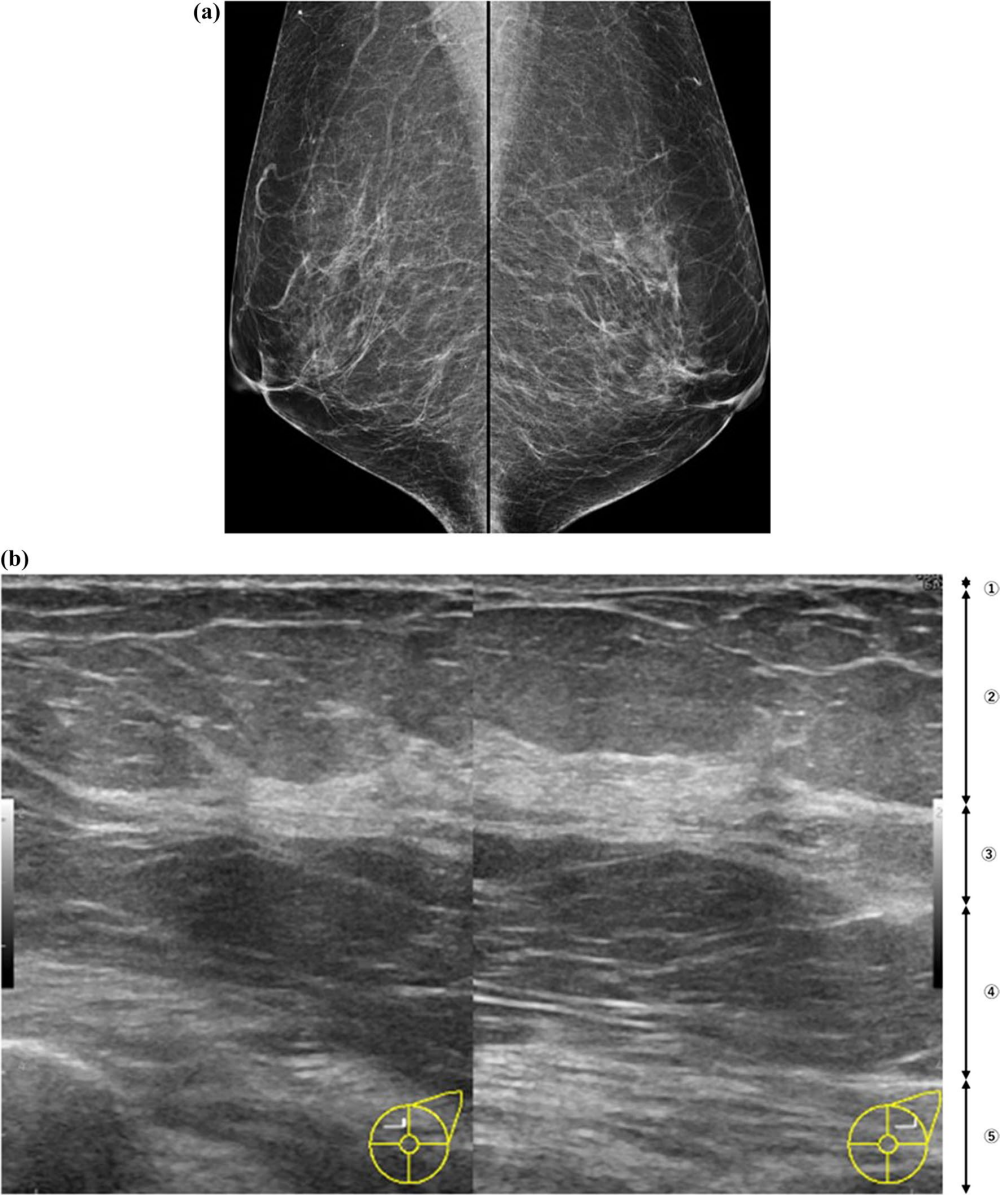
Mammographic nondense breasts that are also ultrasonographic nondense breasts without evident fat lobules in the fibroglandular tissue but with a FGT thickness-to-FAT thickness ratio ≤ 0.93. a Mammograms in bilateral mediolateral oblique view showing scattered areas of fibroglandular density as nondense breasts. b This corresponding ultrasonography image shows a FGT thickness-to-FAT thickness ratio ≤ 0.93 without evident fat lobules in the FGT. 1, skin; 2, subcutaneous fat; 3, FGT; 4, retromammary fat; 5, chest muscle
